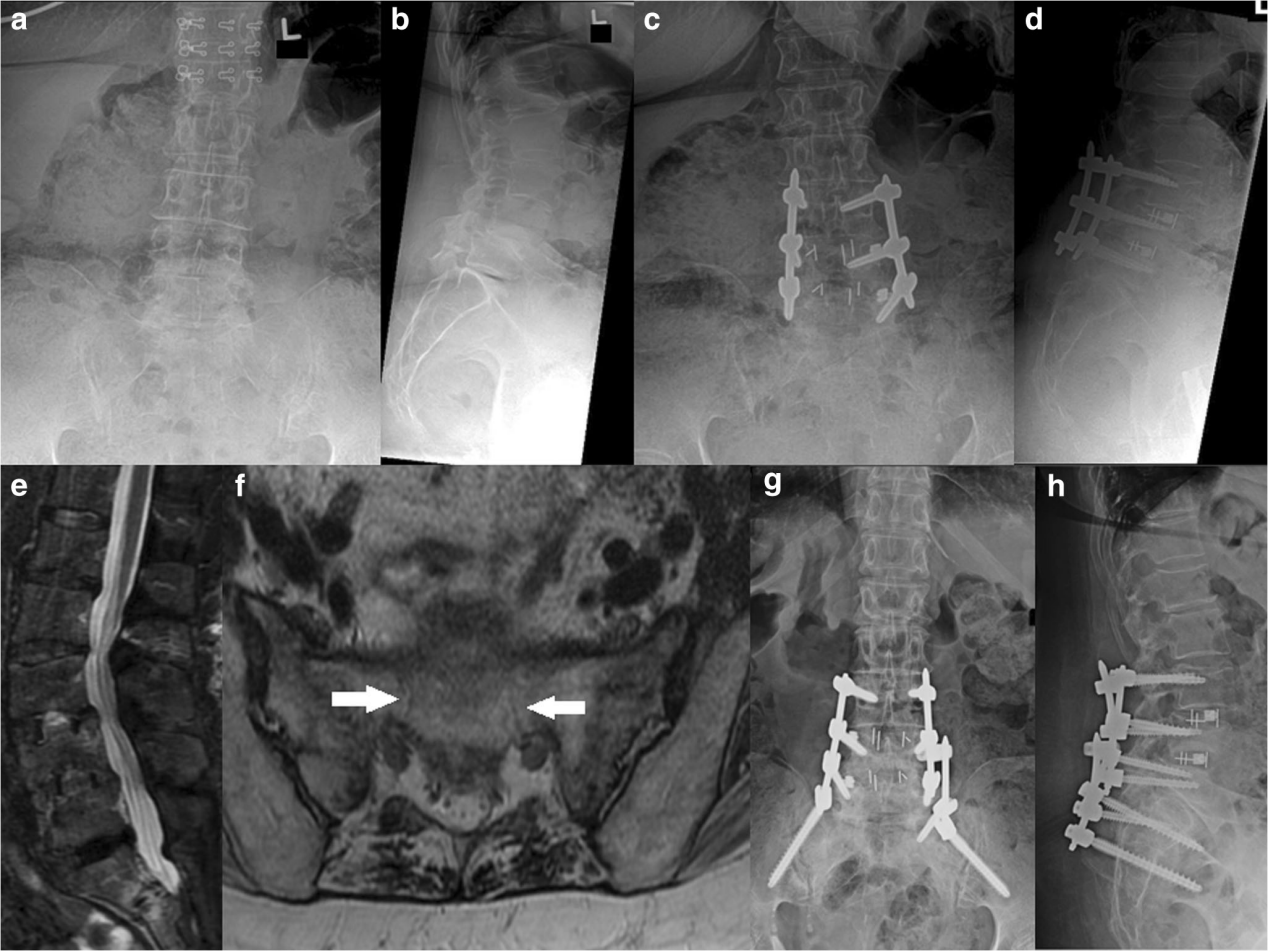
a Anteroposterior (AP) and b lateral plain radiographs taken before index surgery. c AP and d lateral plain radiographs taken after index surgery. e Representative AP and f coronal magnetic resonance imaging cuts showing sacral fractures (white arrows). g AP and h lateral plain radiographs taken after revision surgery.
